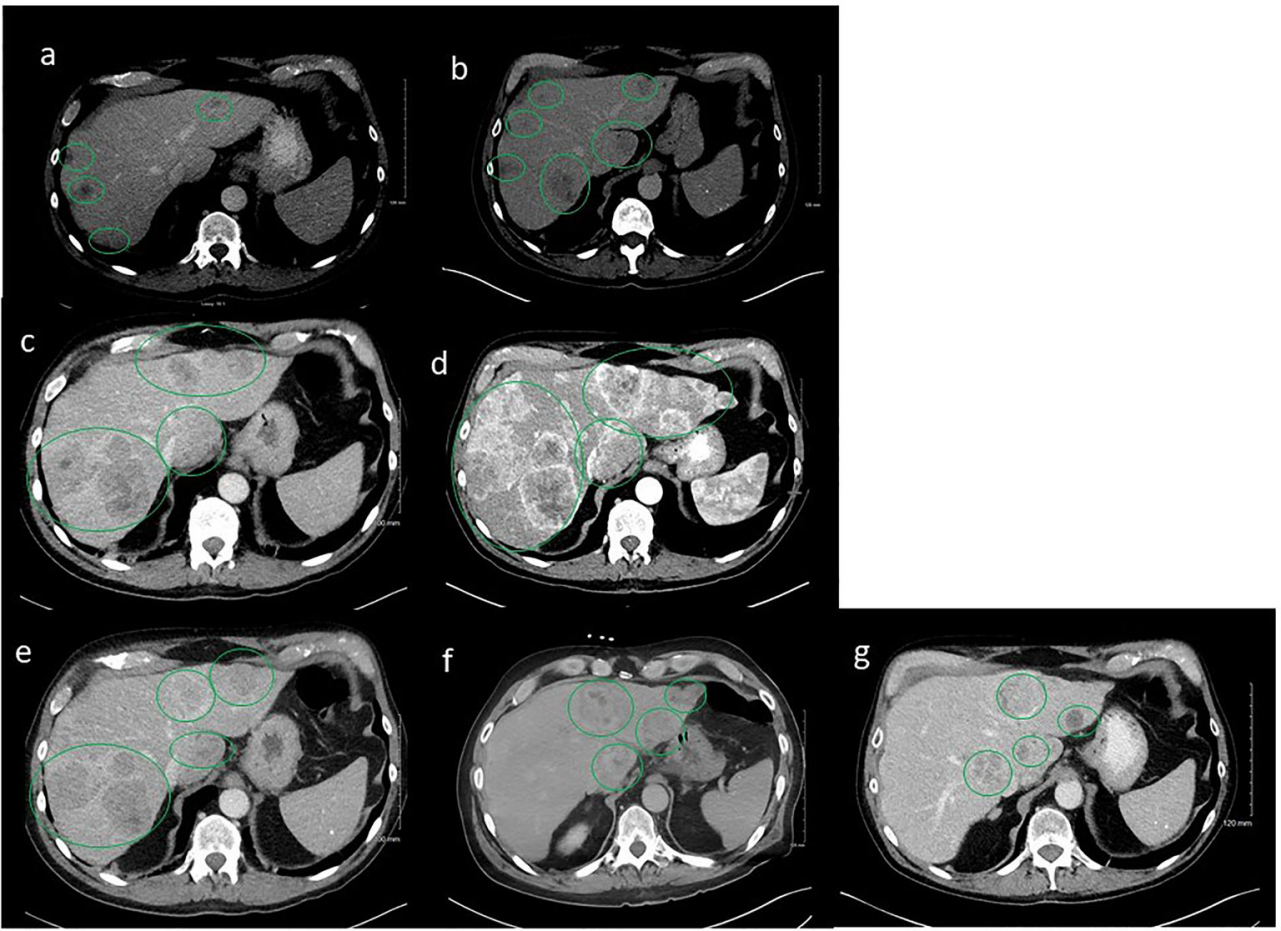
Serial computerized tomography (CT) images of abdomen and pelvis with contrast of the patient’s tumor after sequencing treatments. Green oval cycles represent tumors. (A) Prior to docetaxel, March 2020. (B) Six cycles after docetaxel, June 2020. (C) Two months after enzalutamide, August 2020. (D) After three cycles of cabazitaxel, October 2020. (E) After Y-90 embolization to the right lobe of liver concurrent with carboplatin/abiraterone acetate, December 2020. (F) After Y-90 embolization to the left lobe of liver concurrent with carboplatin/abiraterone acetate, January 2021. (G) On carboplatin/abiraterone acetate, May 2021.
